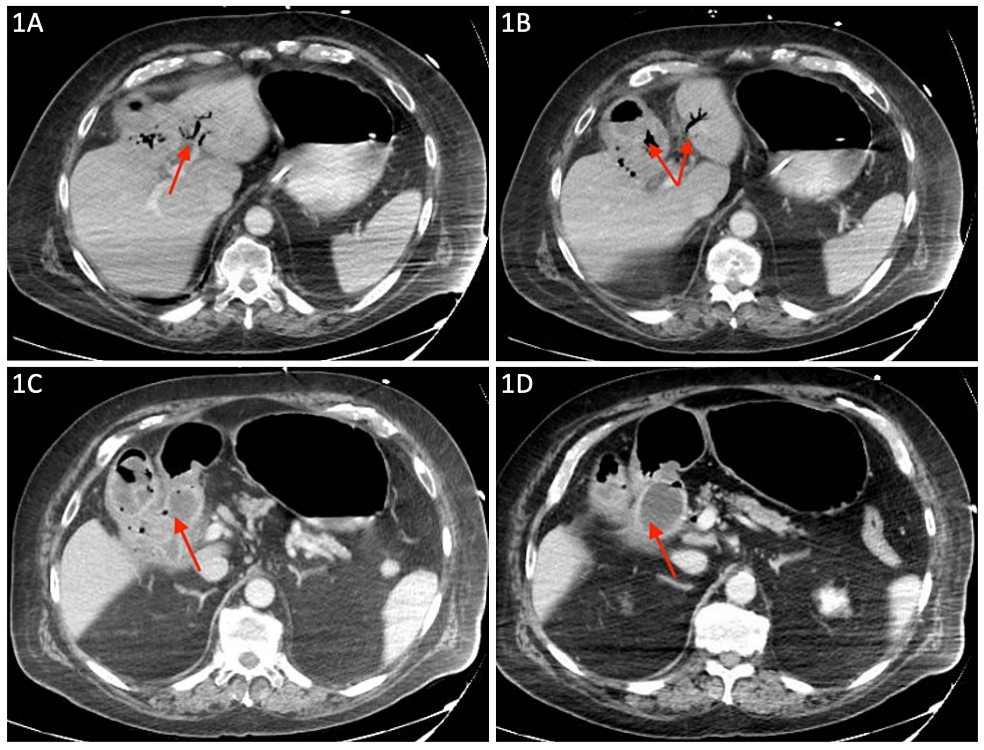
CT of the abdomen/pelvis Pneumobilia is seen in figure (A), with accompanying air in the gallbladder in figure (B). A gallstone in the duodenum and cholecystoduodenal fistula are seen in figure (C), with the full gallstone visualized in the proximal duodenum in figure (D).
